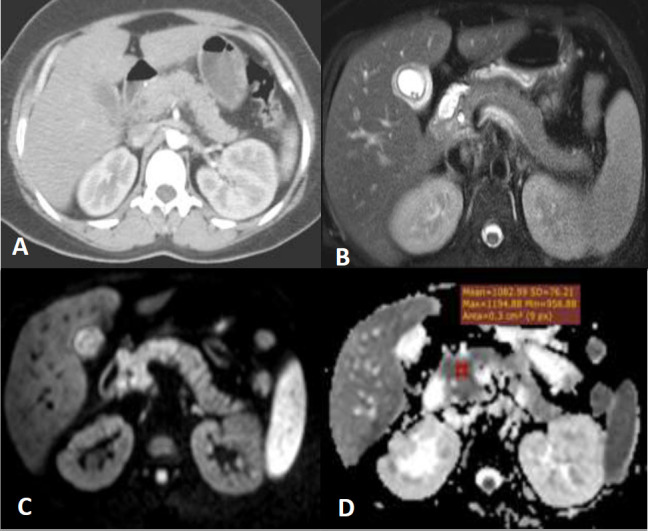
A 35-year-old female patient presented with epigastric abdominal pain radiating to the back and elevated serum amylase. (A) Enhanced CT showed equivocal features for acute pancreatitis. (B) T2WI demonstrated an isointense signal of the pancreatic tissue. (C & D) Show high signal on DWI B800 and low signal on the ADC map, indicating restricted diffusion in the pancreatic head and body. ADC value = 1.082 × 10^−3^ mm^2^/sec. The case was diagnosed as interstitial edematous pancreatitis.
